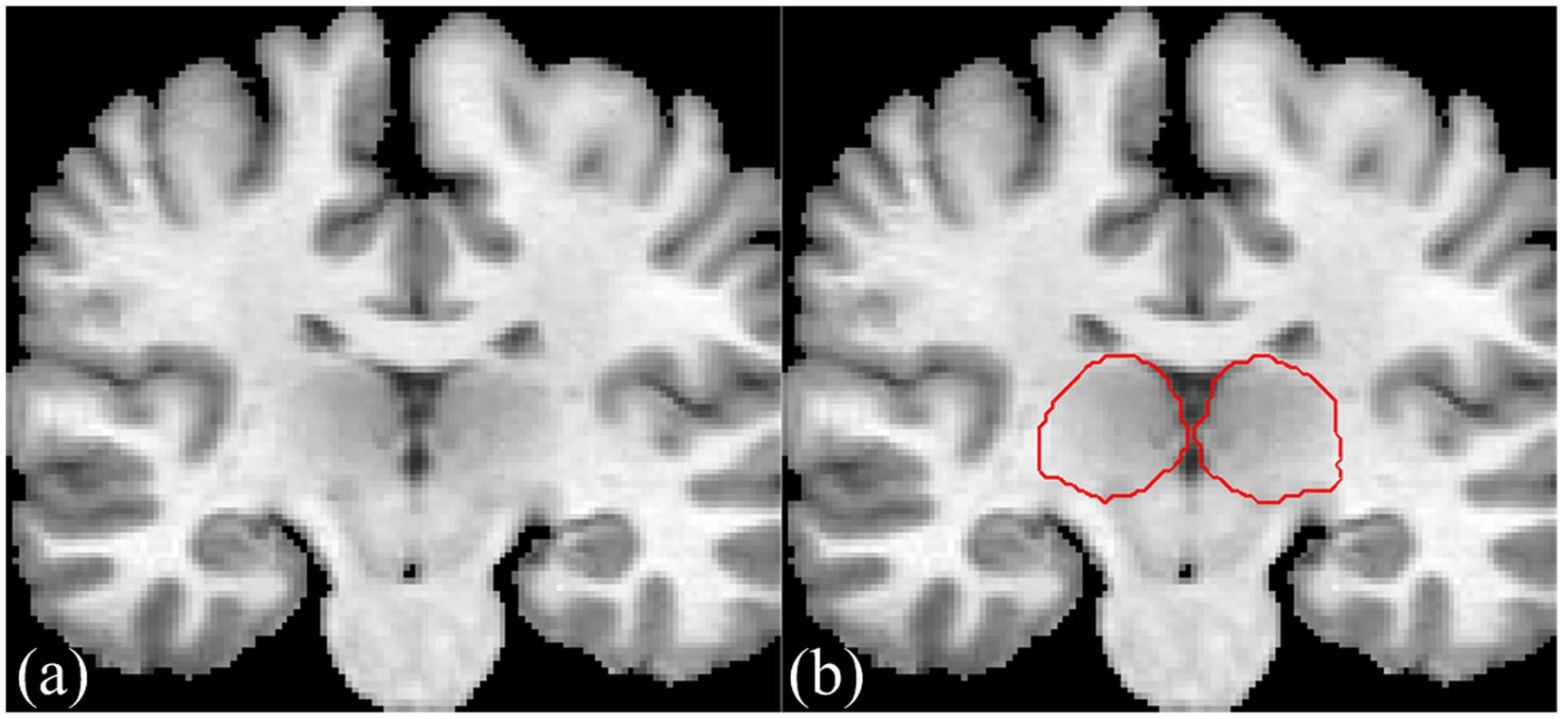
MR T1-weighted image (a) an image slice obtained from a 3-D thalamus MR image. (b) The manually-delineated thalamus boundary shown by the red contour, superimposed on the original image in (a).
